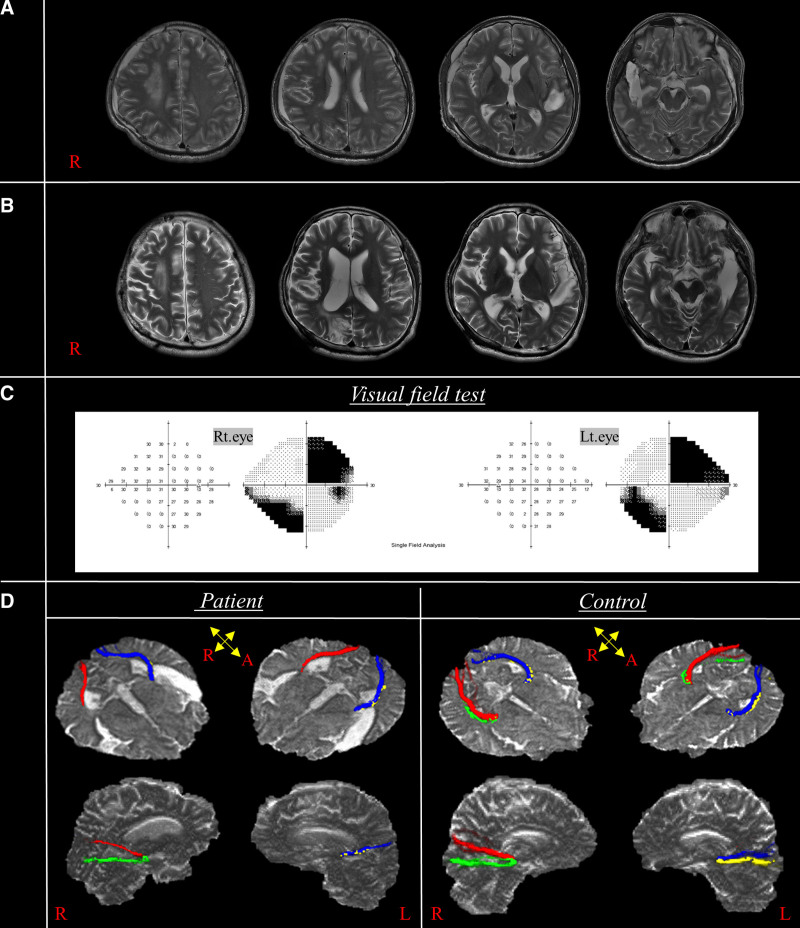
Brain magnetic resonance imaging, diffusion tensor tractography, and visual field test results in the patient. (A) The axial T2 fast spin echo magnetic resonance image taken 2 weeks after injury (June 2013). (B) Axial T2 propeller magnetic resonance image taken 6 years later (July 2019). (C) Automated visual field showing visual field defects in both of the patient’s eyes. (D) Diffusion tensor tractography of the optic radiations in the patient (July 2019) and a control (fiber color: right upper fibers – red, right lower fibers – green, left upper fibers – blue, left lower fibers – yellow).
